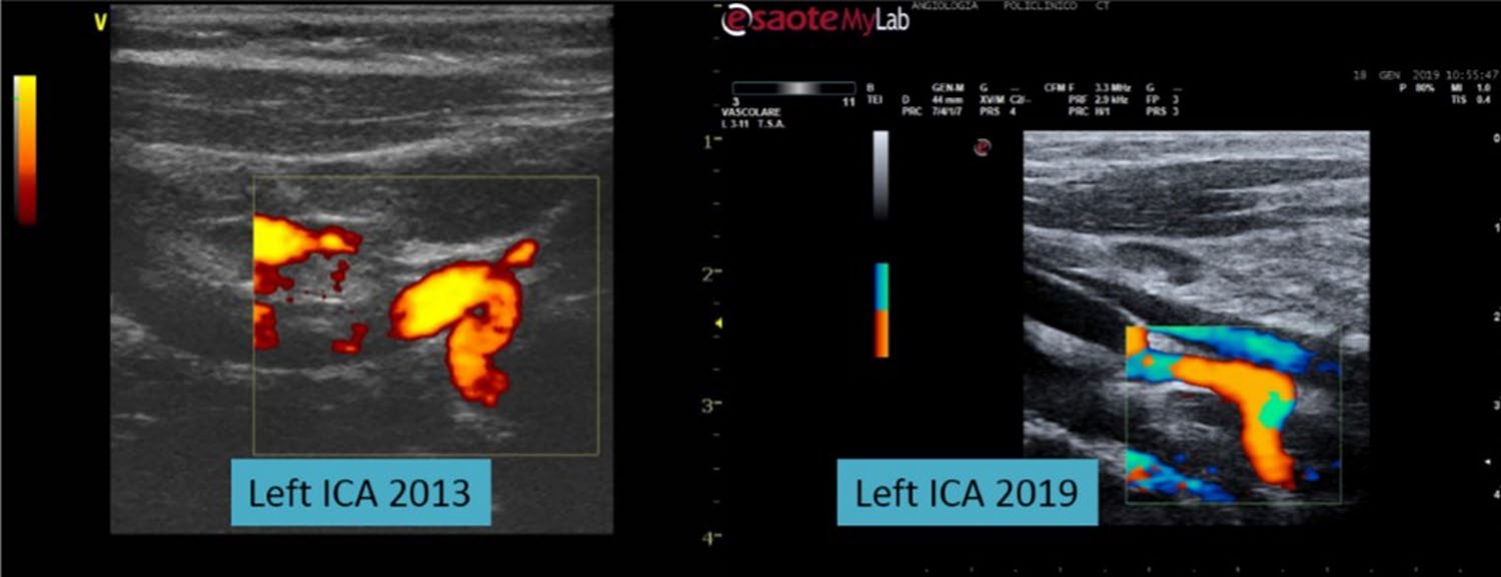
The reduction of the internal carotid artery (ICA) kinking to a simple tortuosity in a child six years after the first echo-color Doppler (ECD) study. This figure shows the image of ECD study of the left internal carotid artery (ICA) of another child in two different times in his life. a At the age of 9 years old (in 2013), he presented a kinking of the left ICA. b Six years later (in 2019, when he was 15 years old), the ECD study of the same subject’s left ICA showed a reduction of his anatomical anomaly to a simple tortuosity with an angulation > 90°
